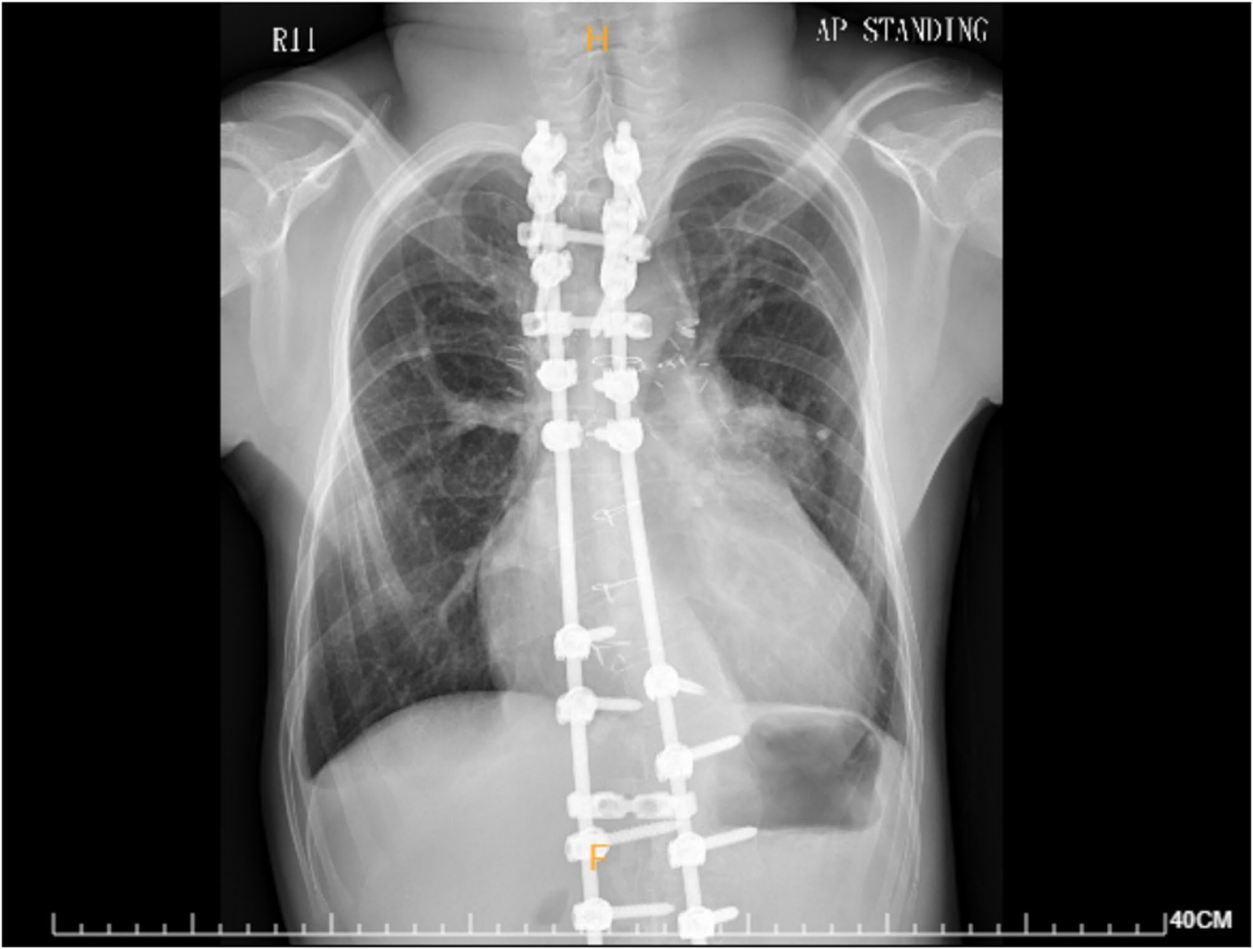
A chest radiograph taken 1 month before the current hospitalization, showing cardiomegaly and spinal rods implanted for correction of scoliosis
